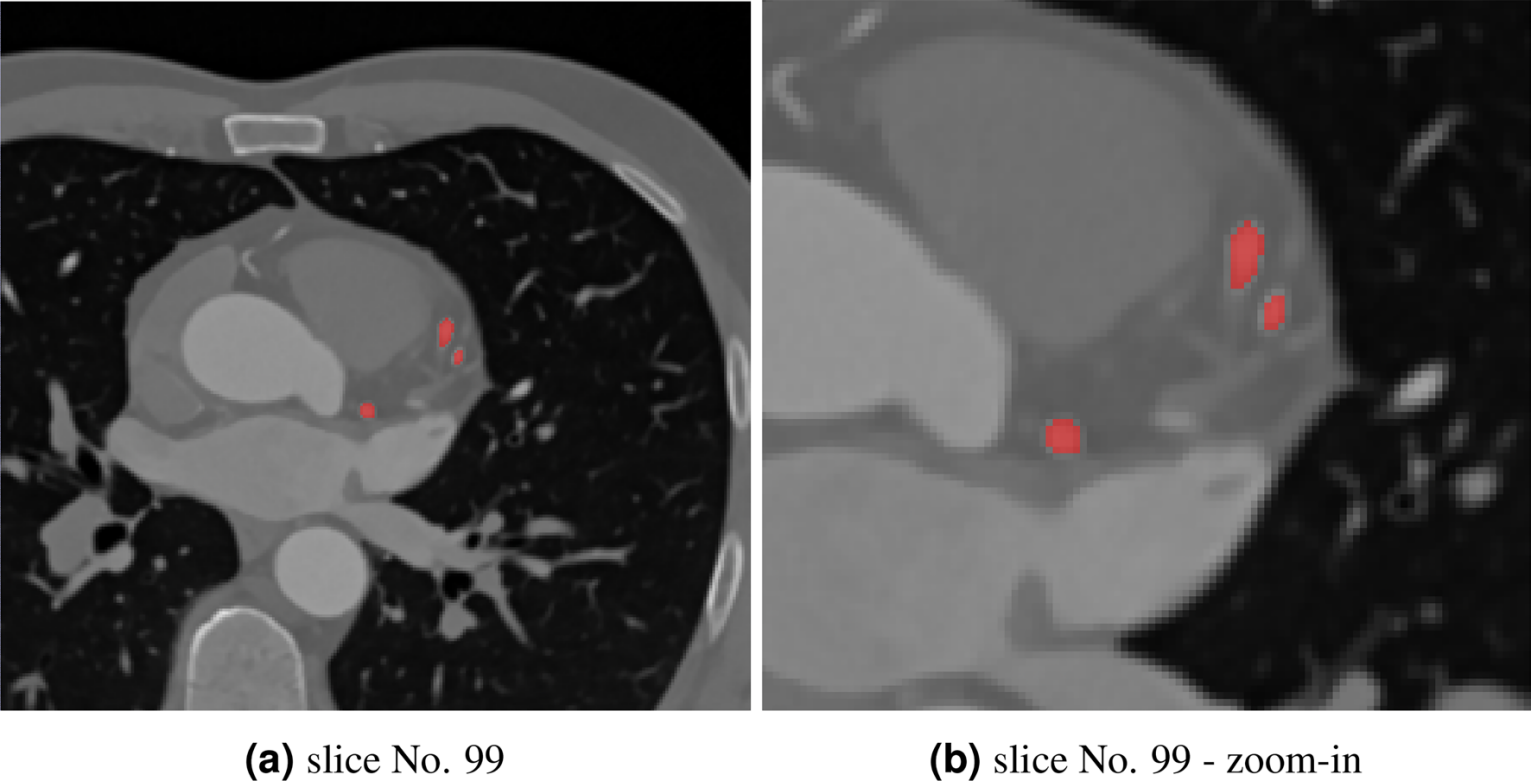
Segmented arteries overlaid on the low-resolution CT images (70% phase of the cardiac cycle). Image (a) shows data from slice No. 99. Image (b) presents a zoom-in on the arteries of interest.
